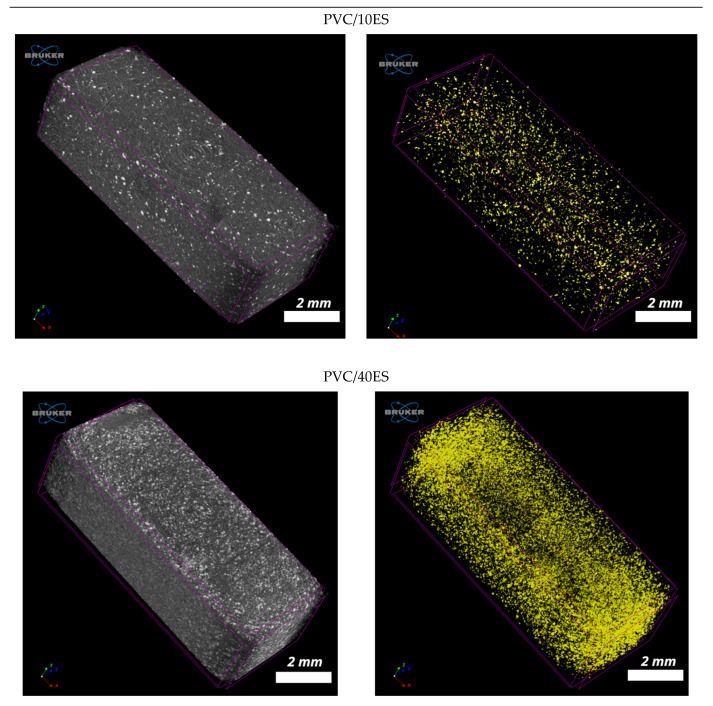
The 3D visualizations of pieces scanned with microcomputed tomography (the left column) and the same pieces after removing the PVC matrix, with ES particles as yellow spots.
